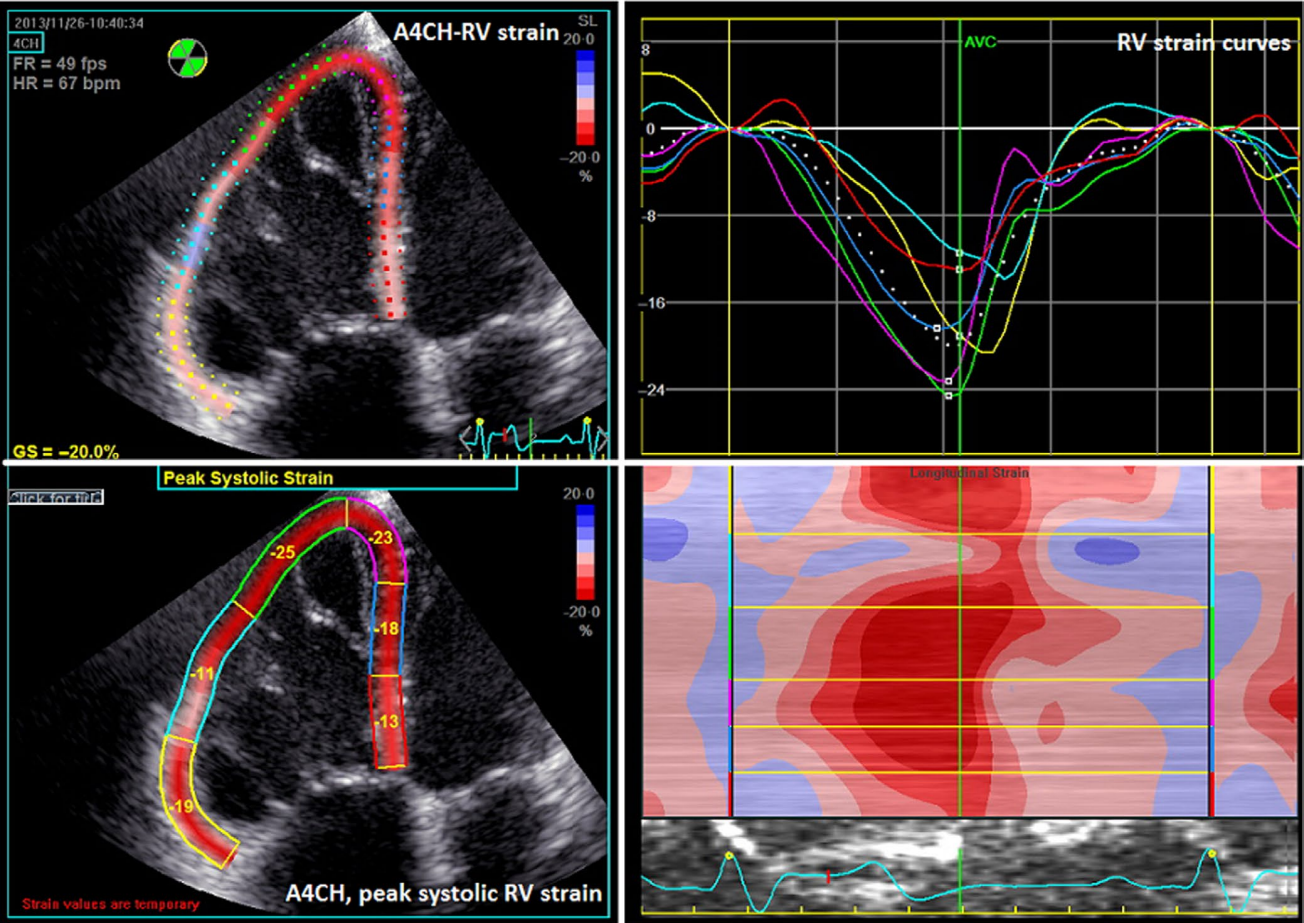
Longitudinal echocardiographic strain analysis of the right ventricle. Longitudinal Strain analysis of the right ventricle (RV). In the first step, the user sets the points in the posterior and septal RV wall at the level of the tricuspid valve and in the RV apex starting the tracking of the RV endocardial border in the apical four‐chamber view (A4CH) (left top). The strain curves are generated by the software (right top). After approval of the endocardial tracking by the user, peak systolic strain values are displayed for all six RV segments and for global systolic RV strain (left bottom). AVC, aortic valve closure
